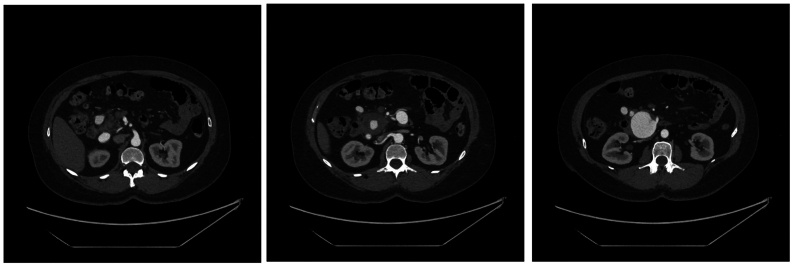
Axial cross sections of abdominal computed tomography (CT) scan with contrast shows multiple mesenteric aneurysms involving branches of the superior mesenteric artery, gastroduodenal artery, and pancreaticoduodenal arcade.
